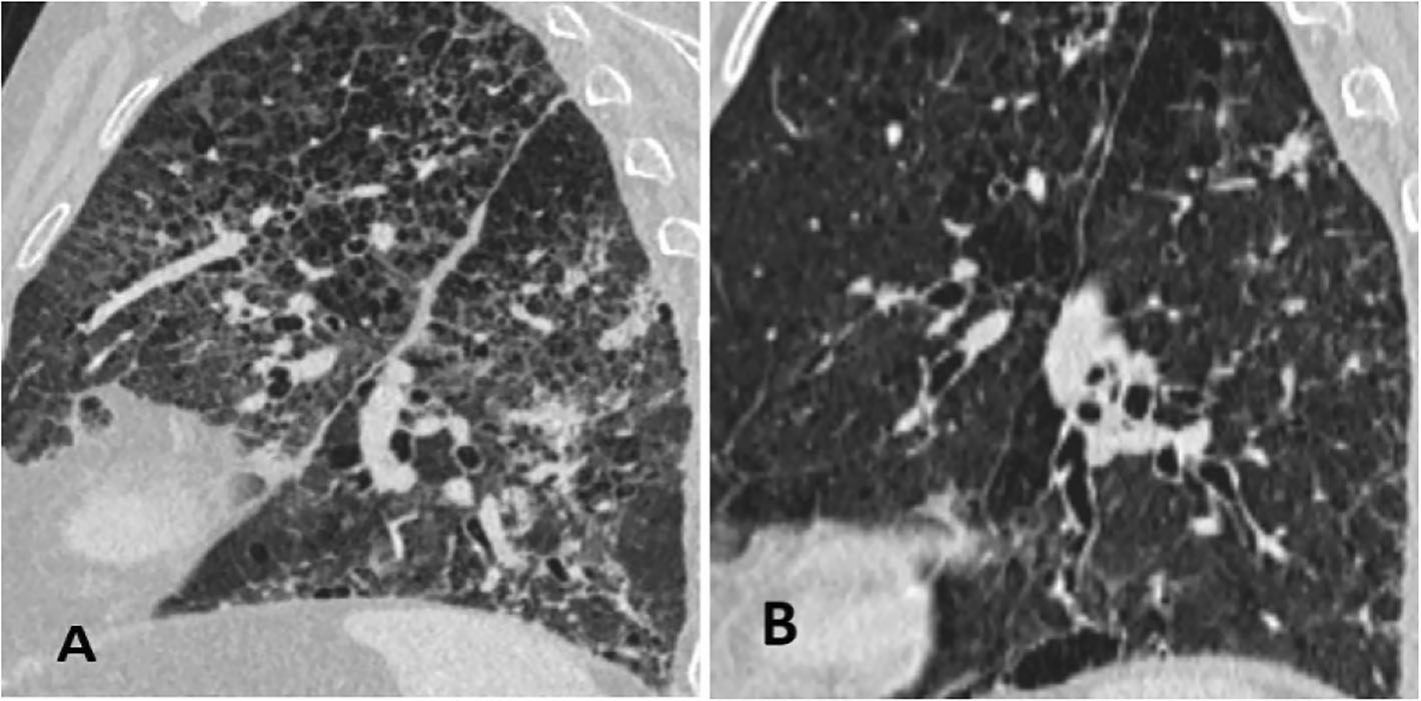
Sagittal reconstruction of chest HRCT before (a) and after (b) the treatment
